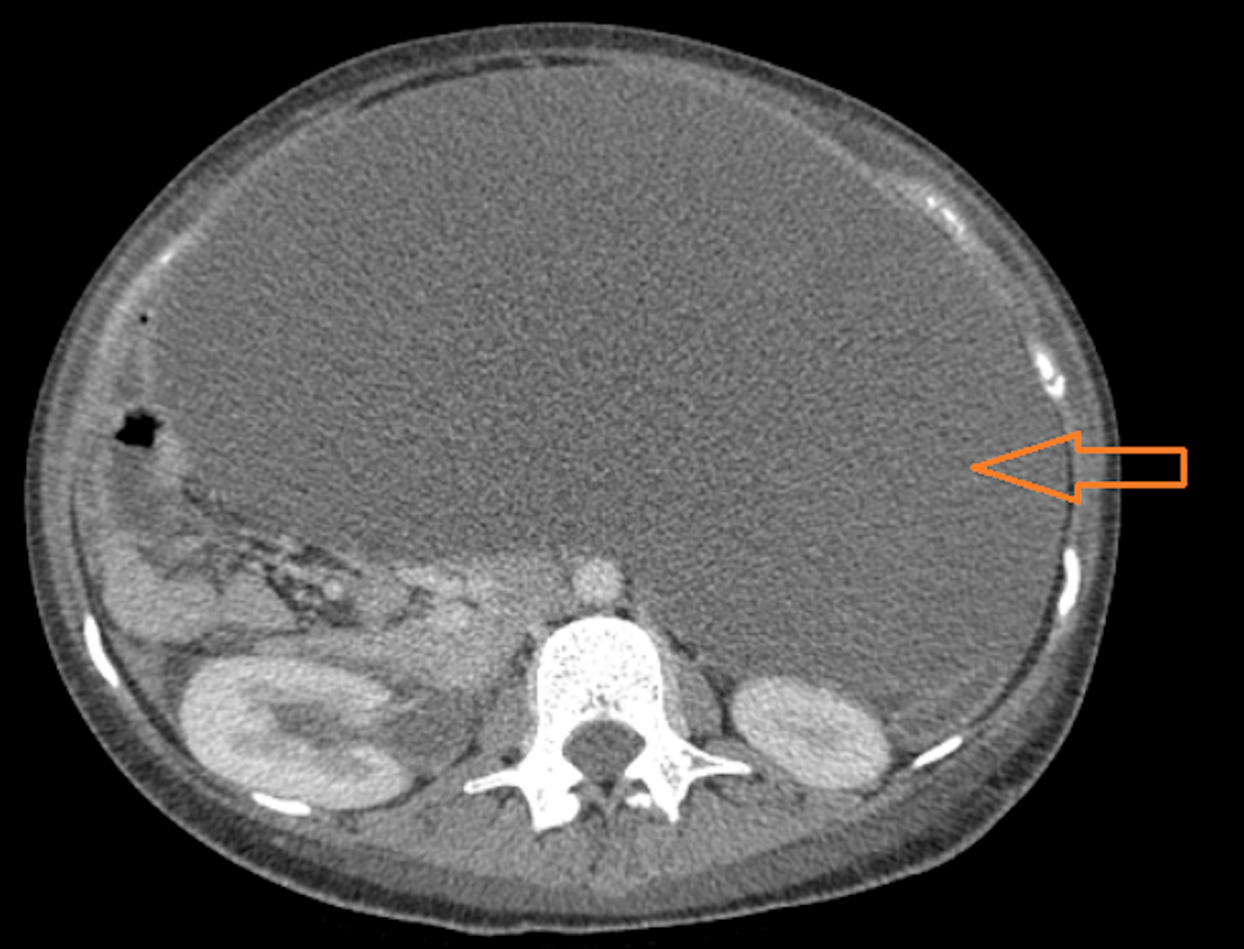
An axial CT scan (abdomen and pelvis) delineating an exorbitant mass (arrow) occupying the entire abdomen and pelvis.
